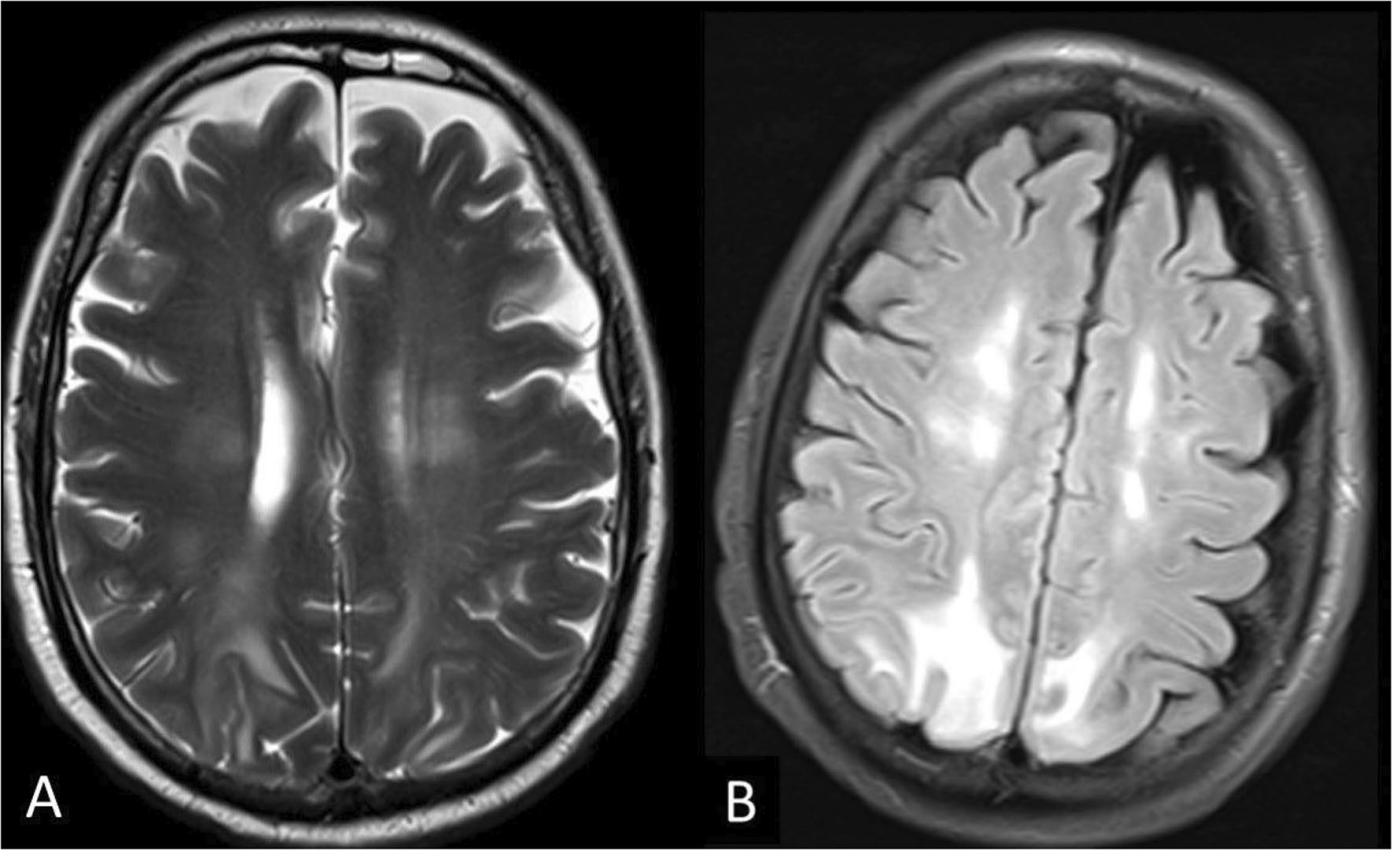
PRES. A 47-year-old man with COVID-19 admitted to ICU, and developed acute hypertension and generalized tonic–clonic convulsions for the first time. a, b Axial T2-weighted and FLAIR image demonstrates bilateral subcortical parieto-occipital white matter hyperintense signal extending to involve superior frontal regions
